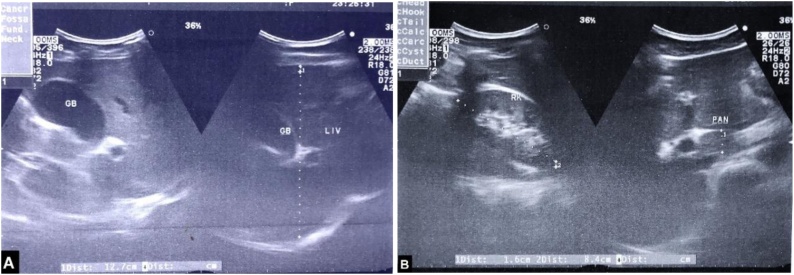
Image-A shows sonographic appearance of liver and gallbladder, image-B shows right kidney and pancreatic regions.
